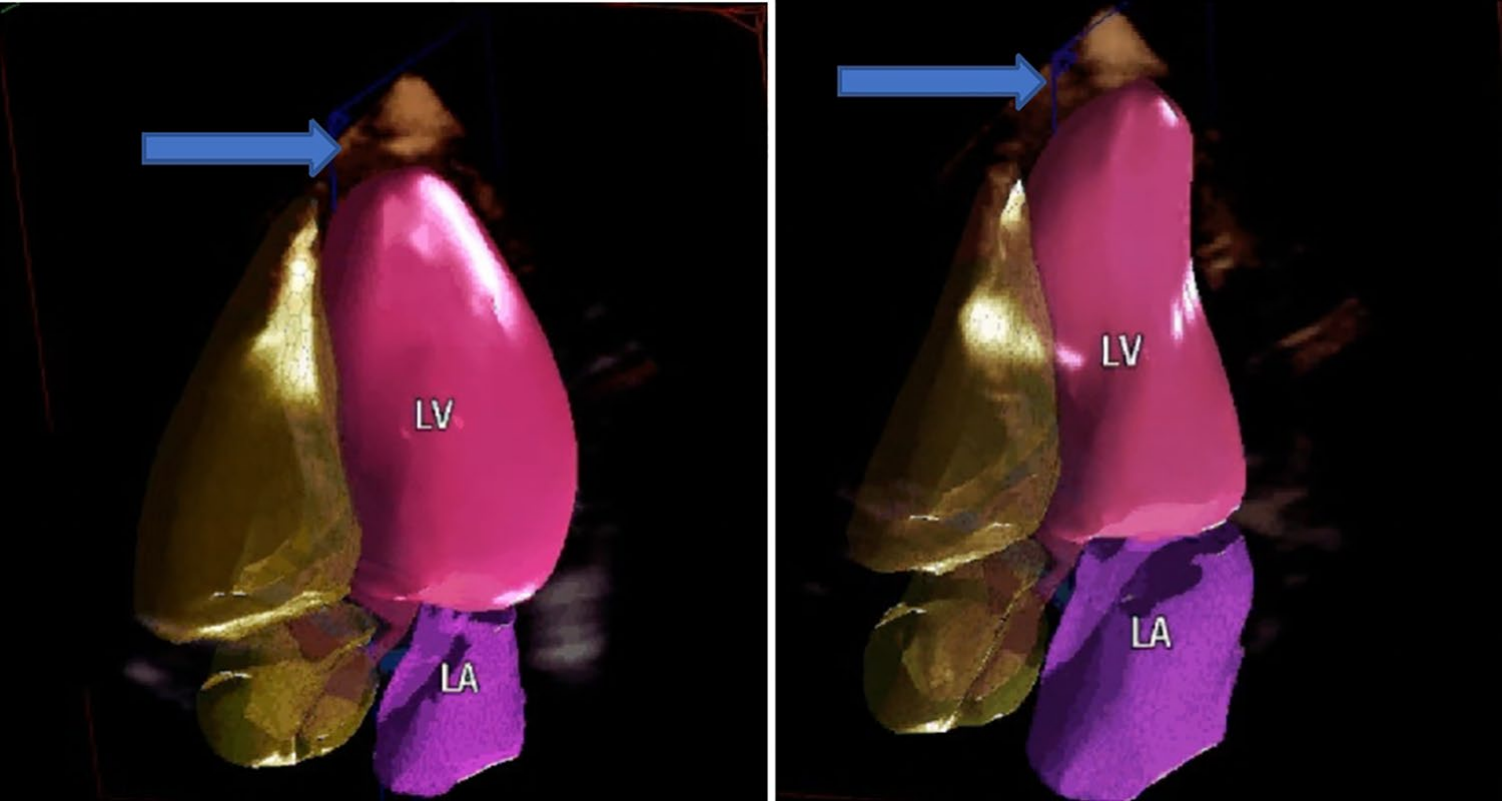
Three-dimensional transthoracic echocardiographic images of dyskinesis seen during diastole (left) and systole (right). Soft tissue overlying the left ventricle (LV) demarcated by arrows with displacement noted at the apex
